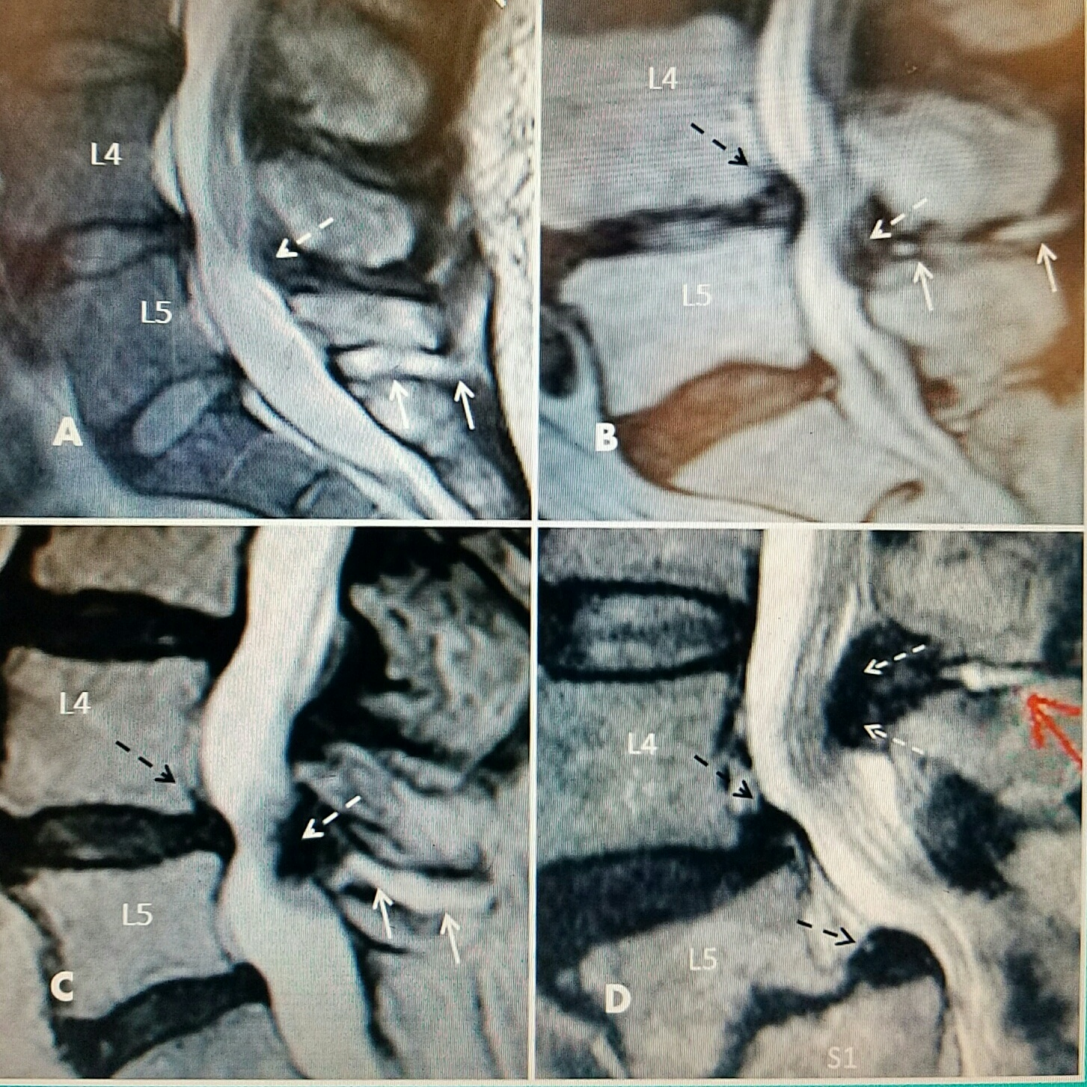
Interspinous fluid with L4-5 one level stenosis on T2 sagittal magnetic resonance imaging (MRI) studies A: L5-S1 large interspinous hyperintense fluid (two solid white arrows) with no ligamentous enlargement. L4-5 minimal ligamentous enlargement posteriorly (dashed white arrow) with early canal stenosis. L4-5 intervertebral disc has early signs of T2 signal desiccation. B: Narrowed and dessicated intervertebral disc at L4-5 with annular bulge (dashed black arrow) with minimal dorsal posterior spinal cyst (dashed white arrow) causing posterior central canal stenosis connected to interspinous fluid (solid white arrows). C: Grade 1 spondylolisthesis at L4-5 (dashed black arrow), posterior fibrous ligamentum flavum hypertrophy (dashed white arrow) and fluid in interspinous space (solid white arrows). D: Degenerated narrowed L4-5 intervertebral disc with annular bulge and grade 1 spondylolisthesis (superior dashed black arrow). Grade 2 spondylolisthesis at L5-S1 with marked endplate degeneration (inferior dashed black arrow). Posterior interspinous cyst (solid red arrow) with marked ligamentous hypertrophy and stenosis at L4-5 (two dashed white arrows). Marked edema and displacement of cauda equina roots.
